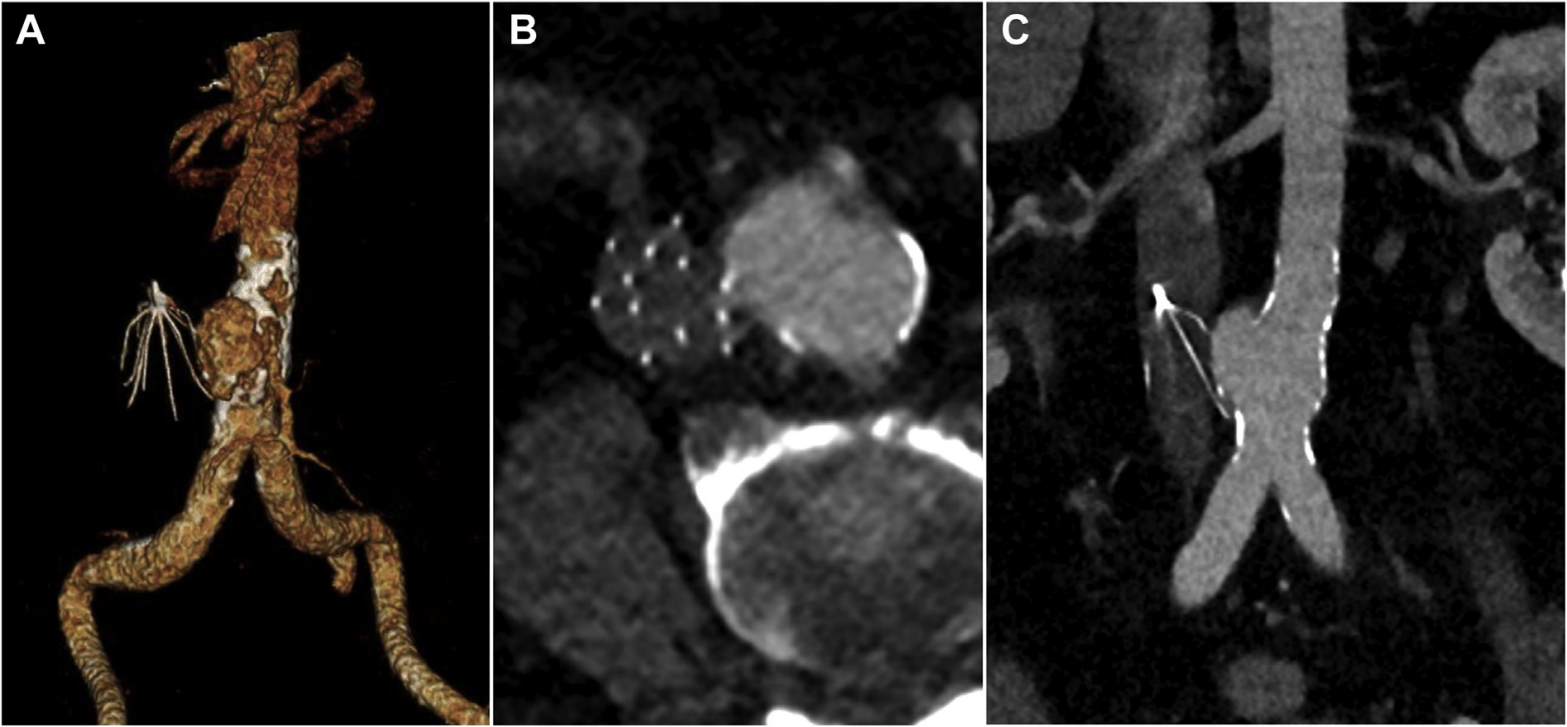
Preoperative computed tomography angiography (CTA). (A) CTA reconstruction, (B) Axial view of the CTA and (C) coronal view of the CTA showing the aortic pseudoaneurysm (APA) and a C.R. Bard Eclipse inferior vena cava filter (IVCF). The struts or prongs of the IVCF extend beyond the margins of the IVC, penetrating the wall of the APA. (B, C) There is a paucity of calcifications involving the pseudoaneurysm, which can be a sign of the aortic wall injury with focal expansion, or pseudoaneurysm formation, leading to separation of the atherosclerotic calcifications. Separation or a focal defect in atherosclerotic calcifications has also been associated with cases of impending aortic rupture. The patient had an extensive infectious workup and had a normal C-reactive protein, negative blood culture, no leukocytosis or fevers and CT findings were not concerning for infection.
